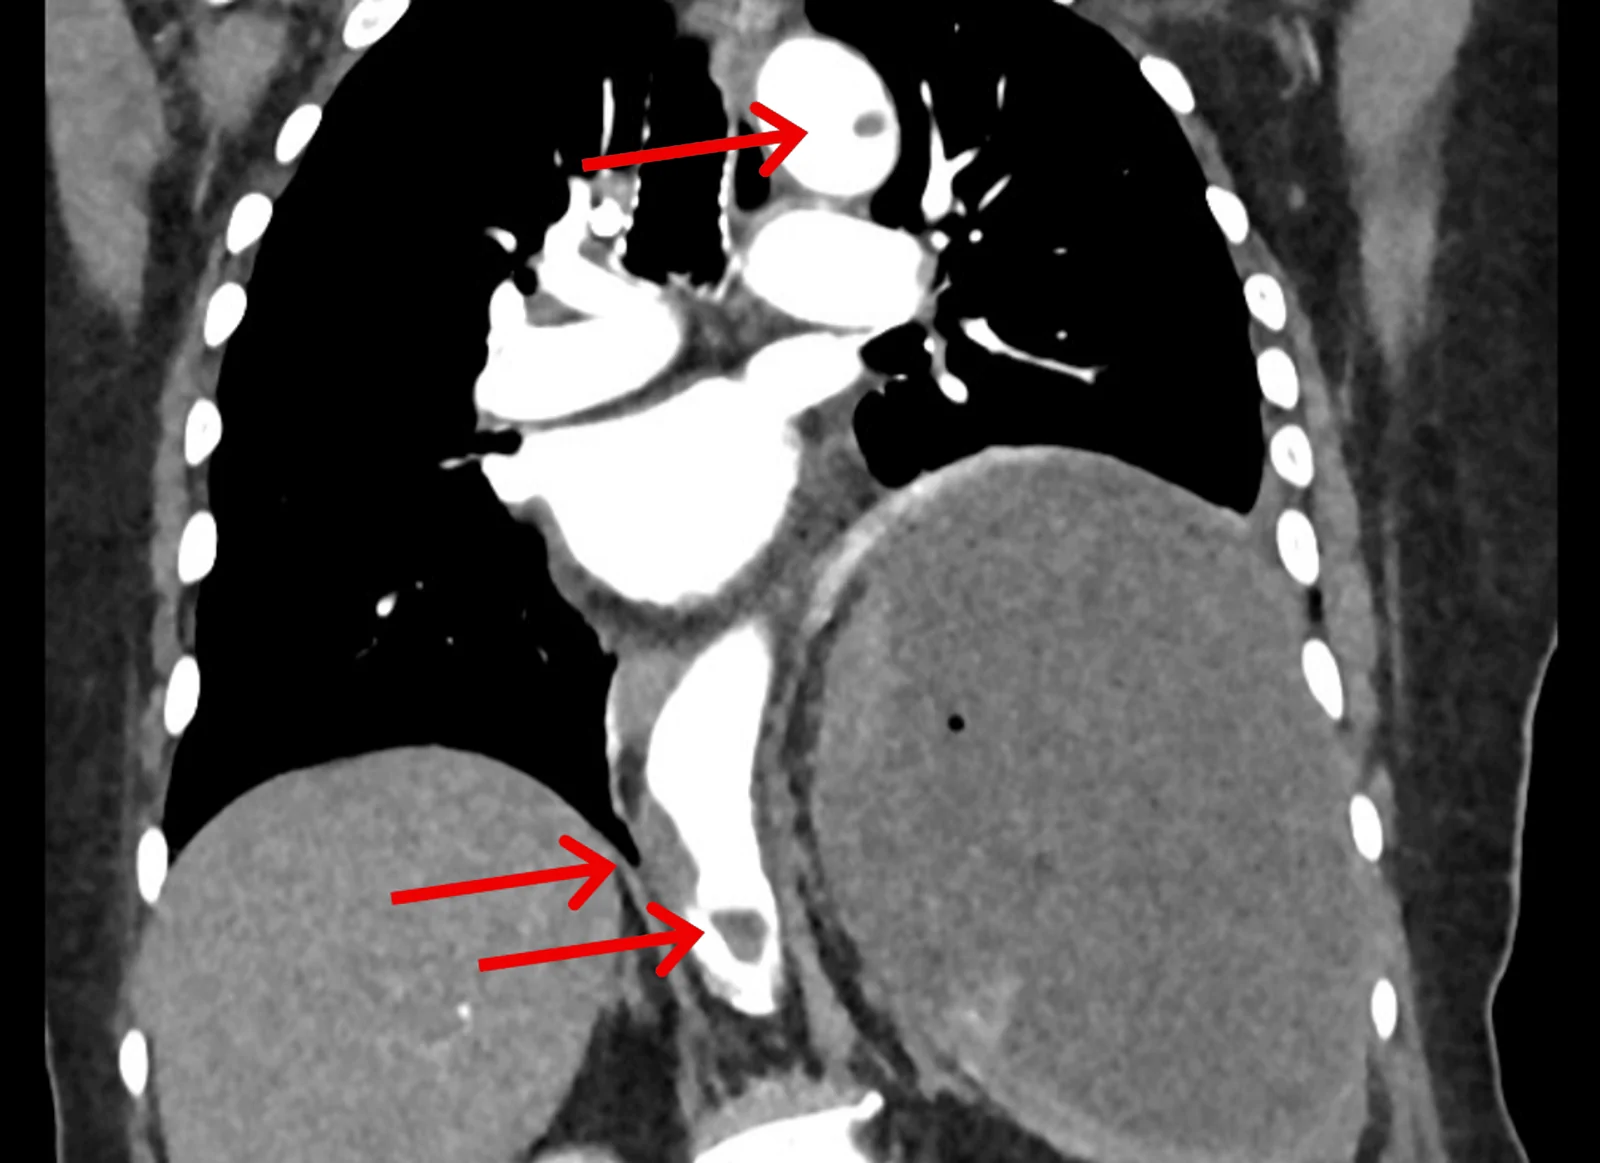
Coronal section of the thoraco-abdominal CT scan in portal phase; multiple atherosclerotic plaques within the aorta indicated by arrows
